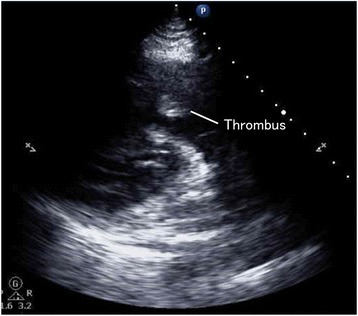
Transthoracic echocardiography. A mobile thrombus is observed in the right ventricle
